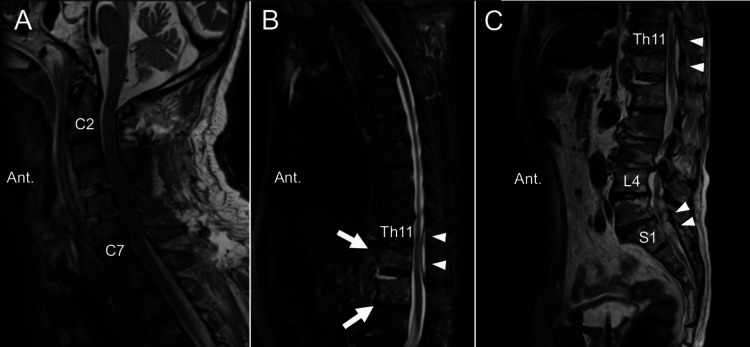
Magnetic resonance images after circumferential cervical fixation. Cervical epidural abscesses, pyogenic spondylitis, and spondylodiscitis resolved after cervical fixation surgery. The persistent epidural abscess at the lower thoracic and lumbosacral levels is identified (white arrowheads). Hyperintensity in the vertebral bodies of Th12 and L1 signifies de novo pyogenic spondylitis due to spread of infection (white arrows)(A: cervicothoracic regions; B: thoracic regions; C: thoracic-lumbar-sacral regions).
